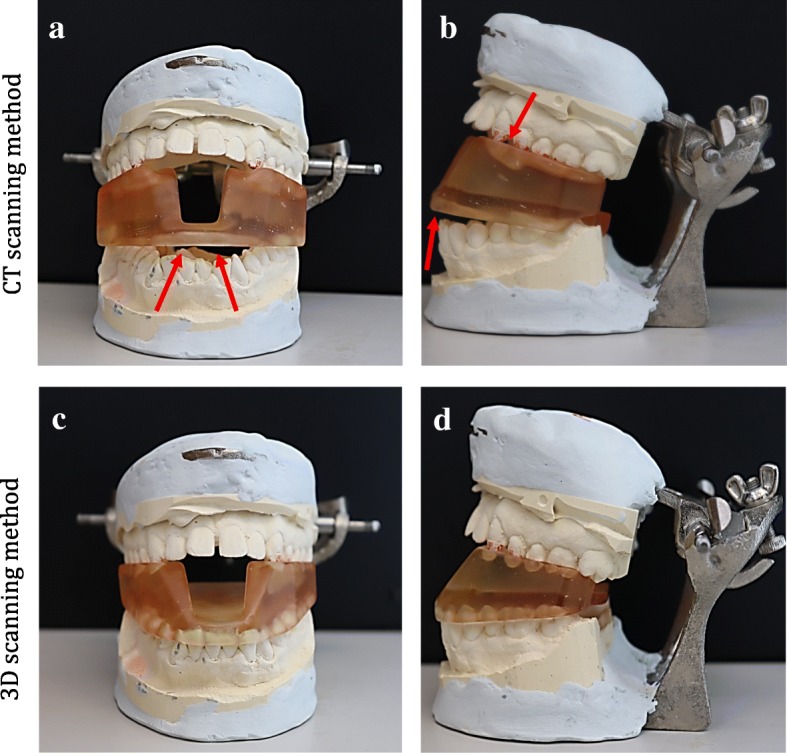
3D-printed stent positioned on the dental stone model of a patient with underbite. a & b Frontal and lateral view of the stent made from CT scan segmentation. Arrows point to areas where the stent does not fit into the model. c & d Frontal and lateral view of the stent made using 3D-scanning method
